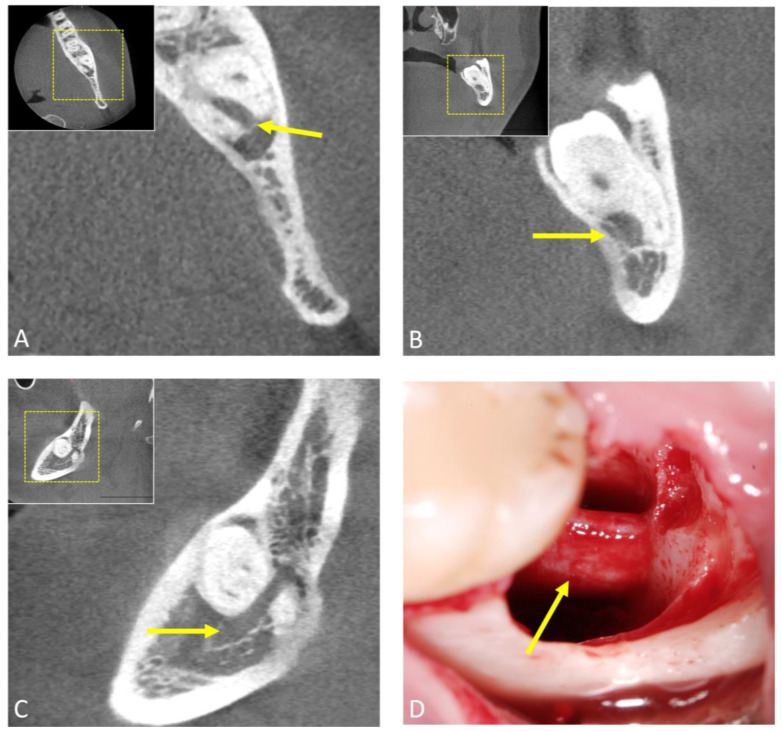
Preoperative cone-beam computed tomography (CBCT) scan of a patient undergoing mandibular third molar surgery. (A) Axial, (B) coronal, and (C) sagittal image reconstructions showing the preoperative high-risk anatomical relationships. The arrow indicates the inferior alveolar canal. Image (D) shows the intraoperative situation after surgical removal of the third molar and the precise anatomical location of the inferior alveolar nerve (arrow).
